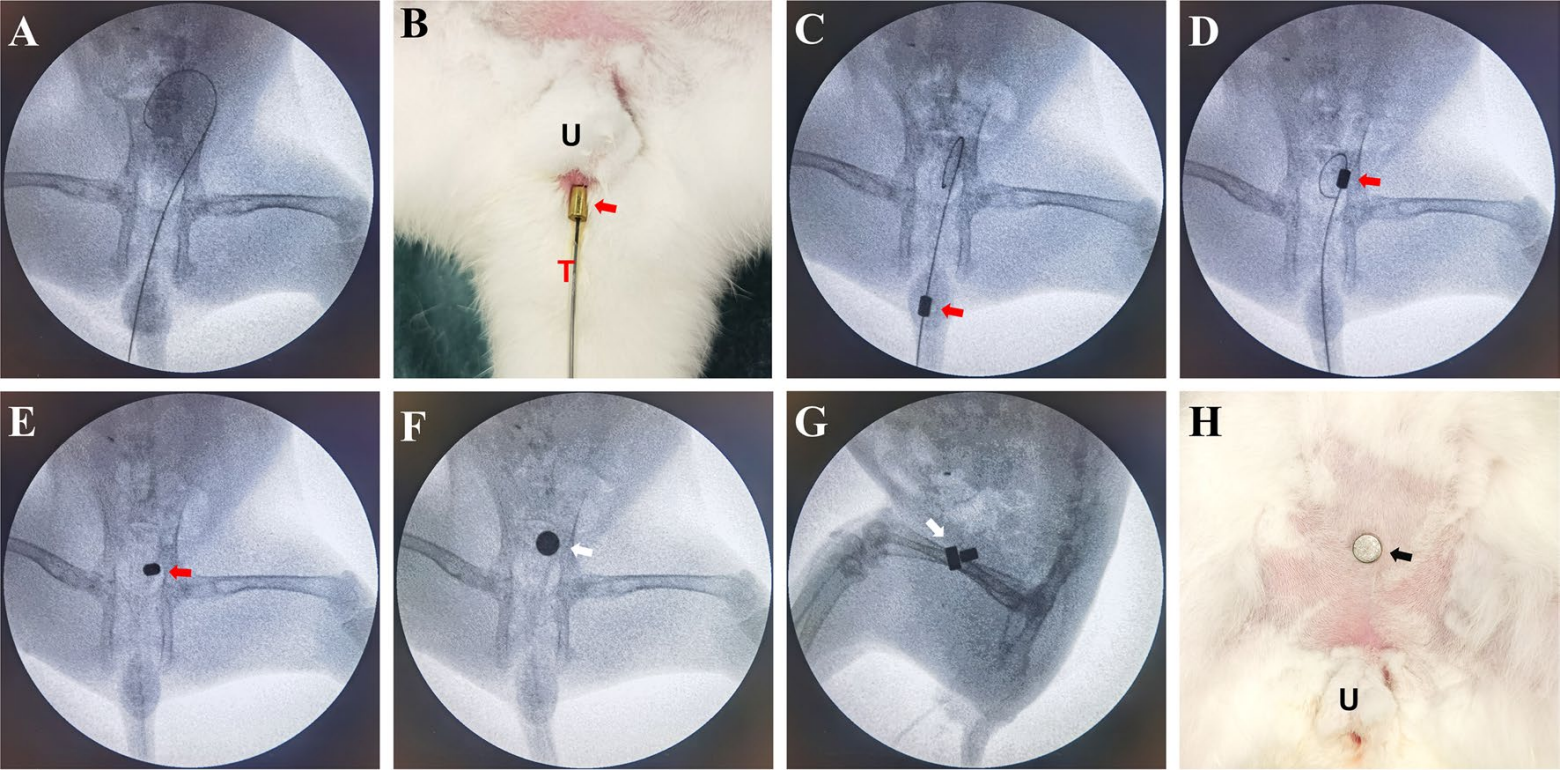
Representative intraoperative images. (A) X-ray image obtained after the guide wire had entered the bladder. (B) Daughter magnet (red arrow) is being inserted through the urethra (U) with the help of a scalp needle tube (T). (C–E) The daughter magnet is inserted into the bladder along the guide wire. (F) Anteroposterior radiograph showing the two magnets attracted together (white arrow). (G) Lateral radiograph showing the two magnets attracted together. (H) Photograph showing the parent magnet (black arrow) on the abdominal wall.
